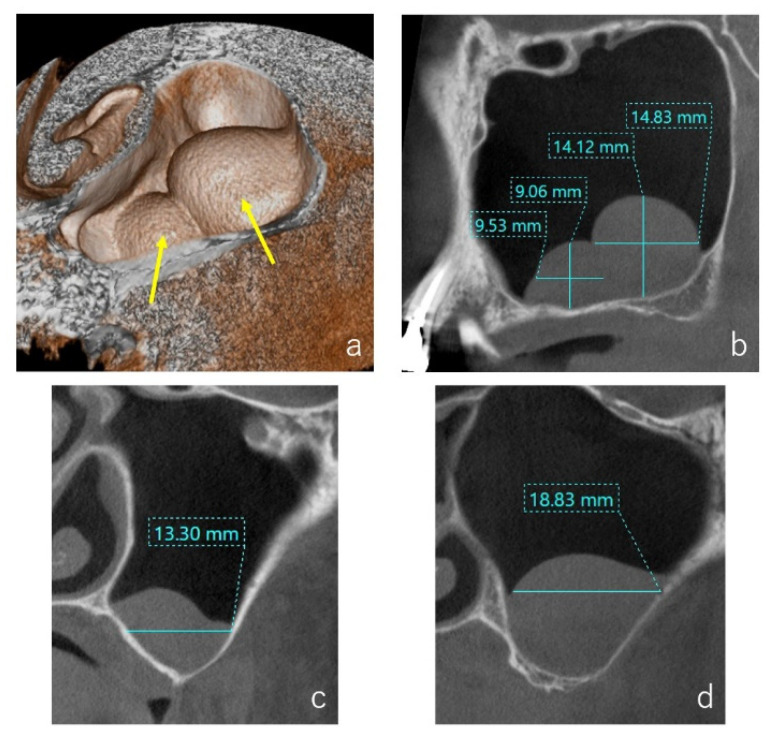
Case: 62 years old female. Two cystic lesions were observed in the left maxillary sinus by the volume rendering image ((a), yellow arrows). A sagittal section of the CT image showed two well-defined ‘dome-shaped’ faintly radiopaque lesions, and the size of mesiodistal and vertical lengths were measured (b). On the buccolingual section of the CT image, the buccolingual length of the medial (c) and distal (d) cystic lesions were measured.
